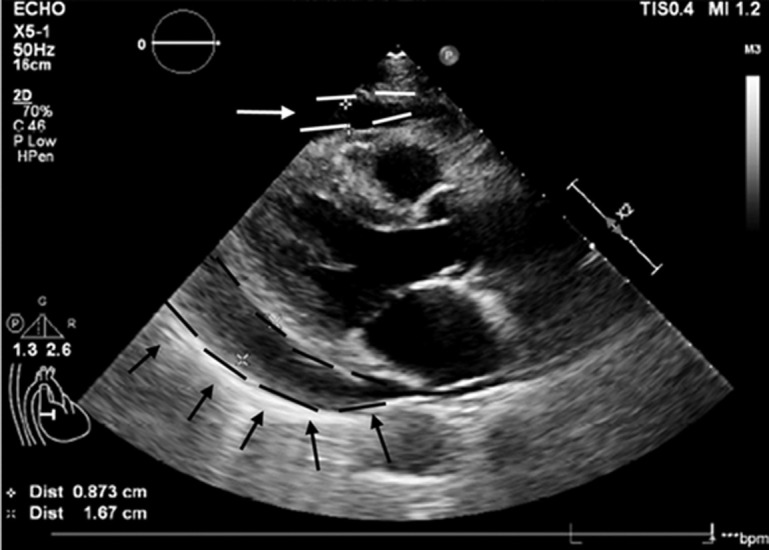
B-scan ultrasonography on admission showing massive bilateral pleural effusion
